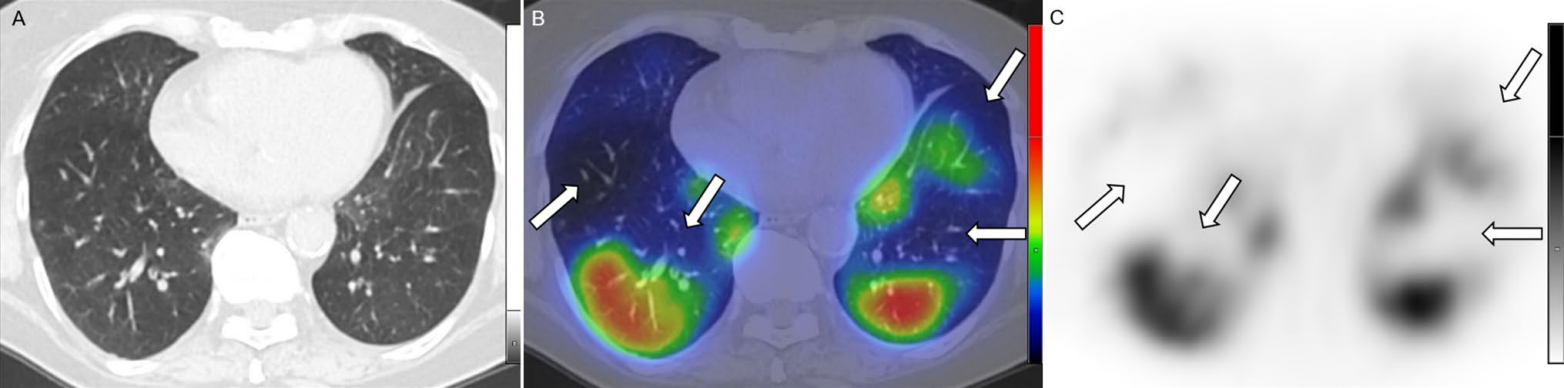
Axial low-dose CT (a), fused Q-SPECT/CT (b), and SPECT images (c) of a COVID-19 patient with dyspnea. A representative figure of bilateral segmental and subsegmental perfusion defects (demonstrated with white arrows) without any ventilation abnormalities. No pulmonary opacities could be depicted in this case (total CT score was 0). *CT* computed tomography, *Q-SPECT/CT* perfusion single-photon emission computed tomography/computed tomography, *COVID-19* coronavirus disease 2019
